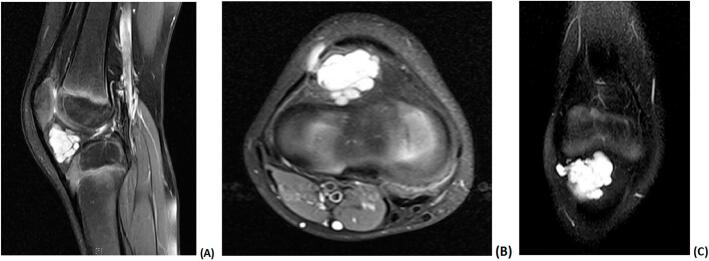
(A), (B) and (C) T1 magnetic resonance imaging of the right knee revealing a hyperintense multilocular cyst suggestive of an intraarticular ganglion cyst in the infrapatellar Hoffa's fat pad with focal extension to the subcutaneous fat via the lateral aspect of the joint capsule and lateral patellar retinaculum.
